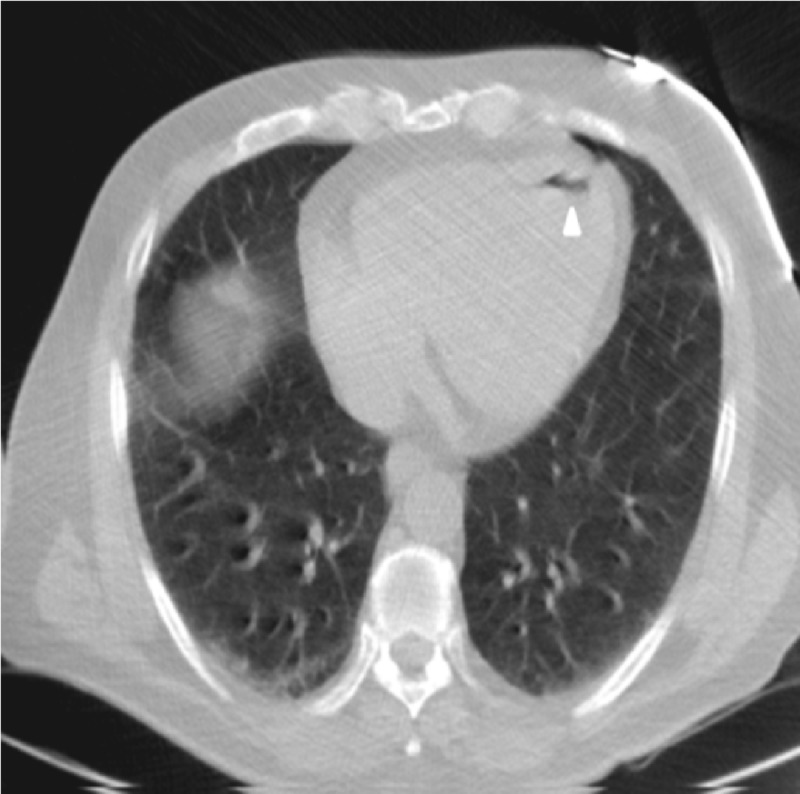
Computed tomography scan of the chest showing air in the left ventricle (arrowhead).
